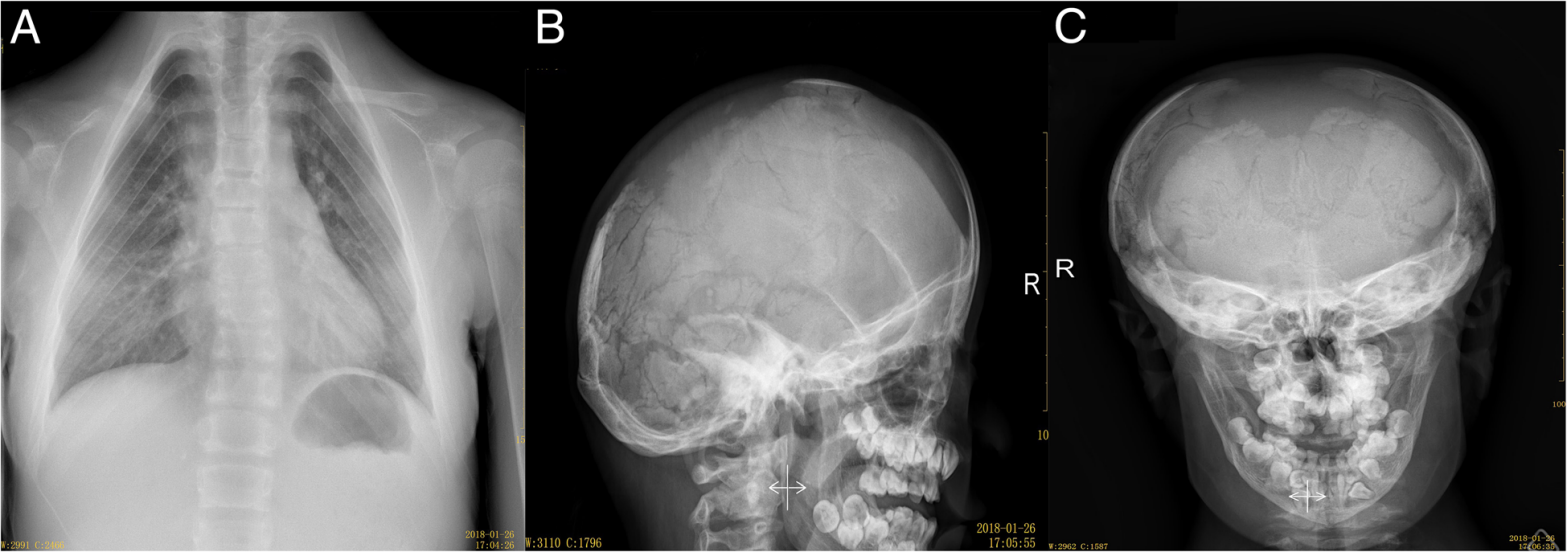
Plain radiographs showing multiple skeletal abnormalities. a: chest radiograph showing bilateral asymmetry clavicles with partial absence of the right; b & c: anteroposterior and lateral cephalograms showing the broad sagittal and coronal sutures, delayed closure of the anterior fontanel, numerous wormian bones, hypoplastic maxilla and unerupted permanent teeth
